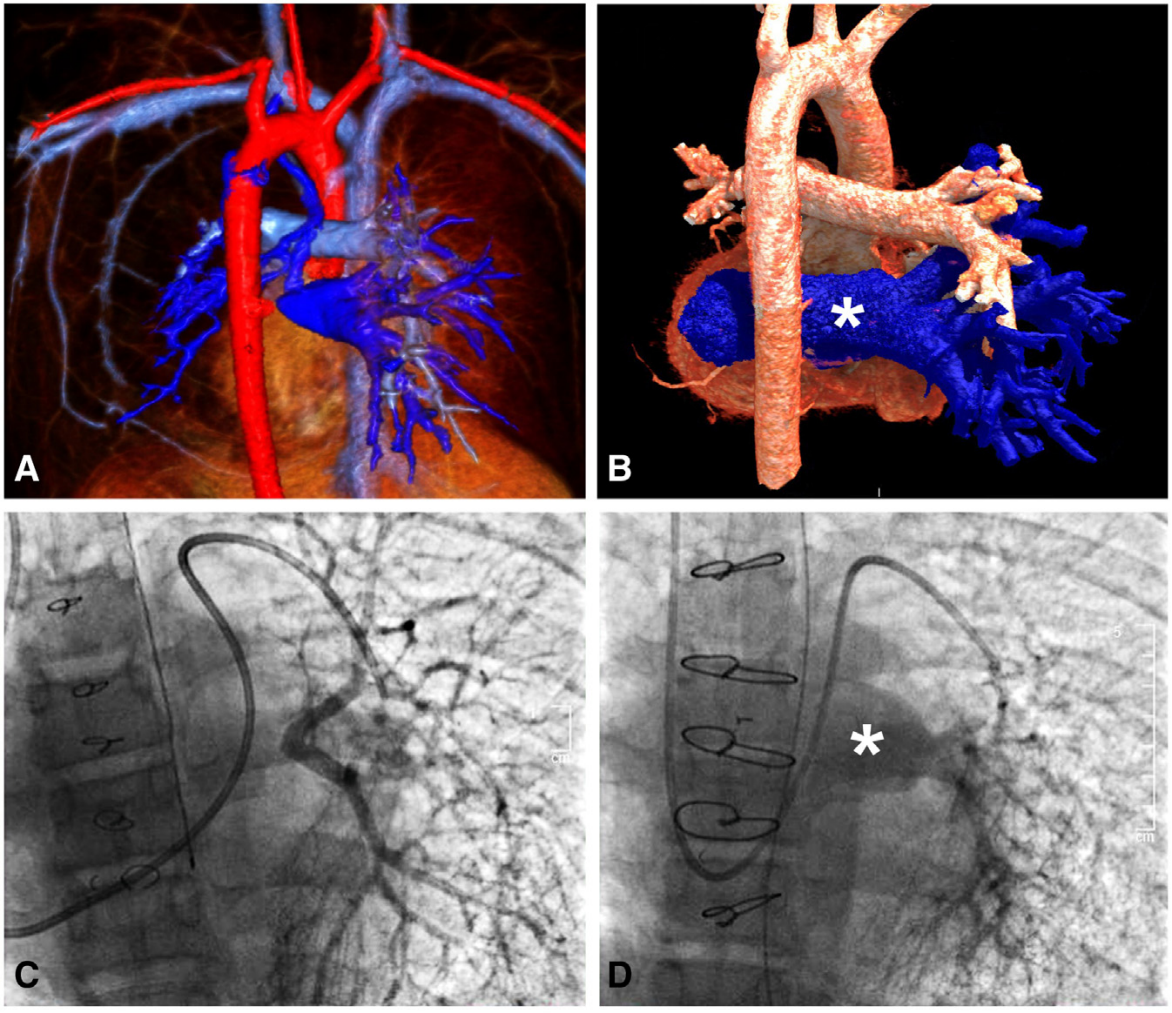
Case 1. A, Preoperative magnetic resonance imaging viewed from the posterior demonstrating the left superior and inferior pulmonary venous tributaries (*blue*) converging to a loose network of collaterals around the left hilum and peribronchial region. B, Postoperative computed tomography scan of the chest, 3-dimensional reconstruction, highlighting the pulmonary homograft (*blue*). C, Preoperative angiography demonstrating left PVO and D, postoperative angiography of left pulmonary veins and patent homograft (∗).
